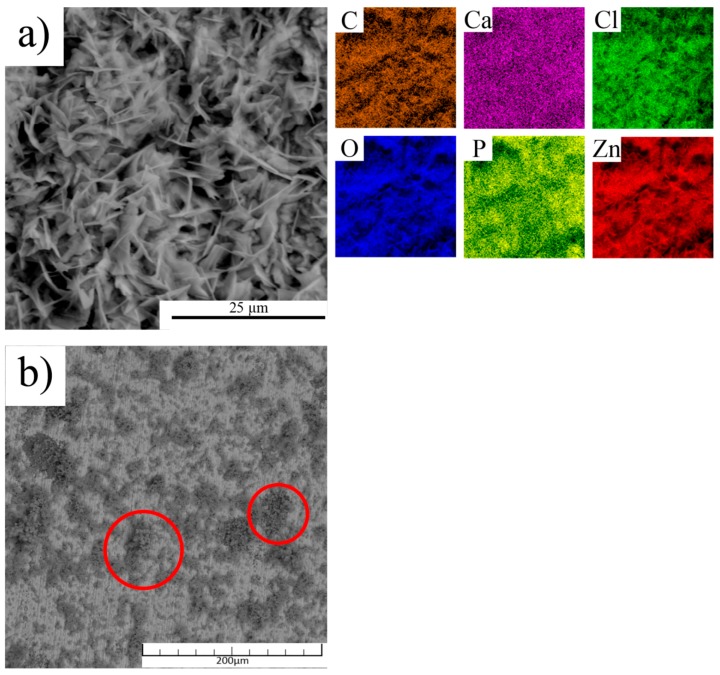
(a) The SEM image and X-ray elemental maps of corrosion products occurred predominantly on the upper layer and (b) SEM image showing the precipitation HA on the HA particles.
